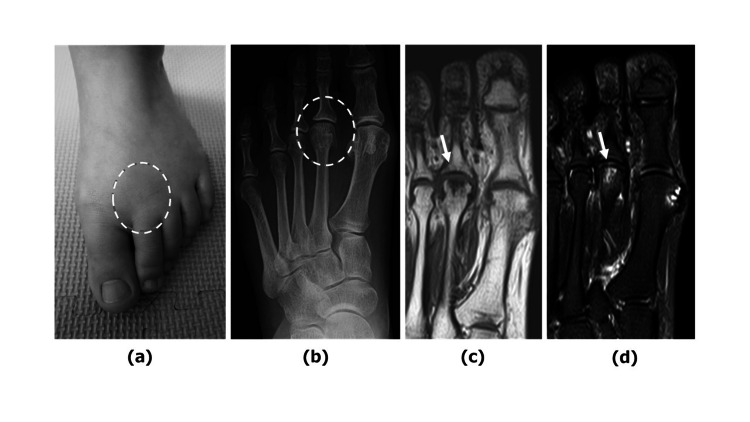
Case 1—Preoperative examination (a) Clinical photograph taken during the initial examination (dotted circle highlights the area of tenderness) (b) AP view radiograph demonstrating flattening deformity of the second metatarsal head (indicated by a dotted circle) (c) MRI T1-weighted image showing low signal in the second metatarsal head (indicated by an arrow) (d) MRI fat-suppressed T2-weighted image showing high signal in the second metatarsal head (indicated by an arrow) AP: Anteroposterior
